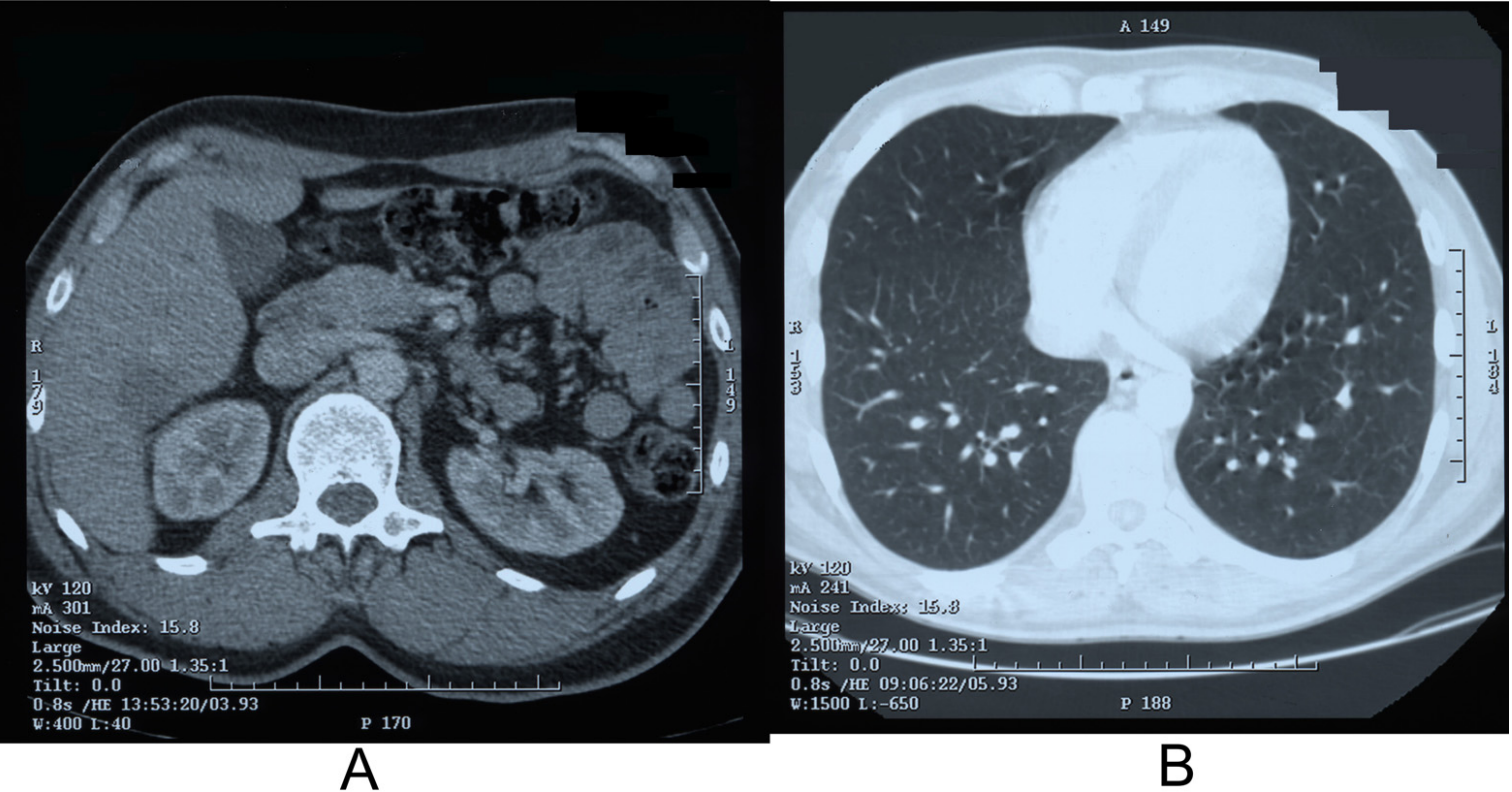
Computed tomography after chemotherapy A – regression of focal changes in liver is visible B – regression of metastases in lungs is visible.
